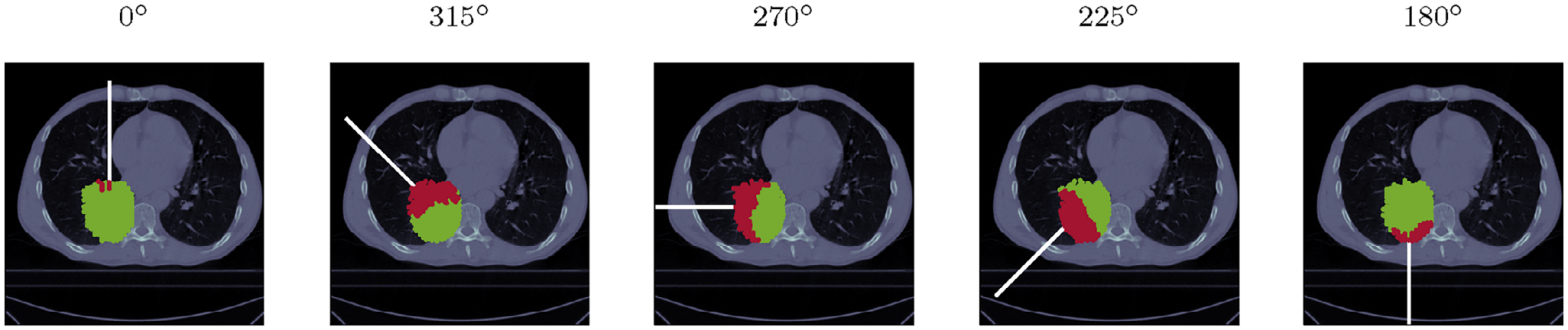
Axial CT slice for patient No.114 for different gantry angles. The overlay highlights the spots with sufficiently large residual helium range in green (
